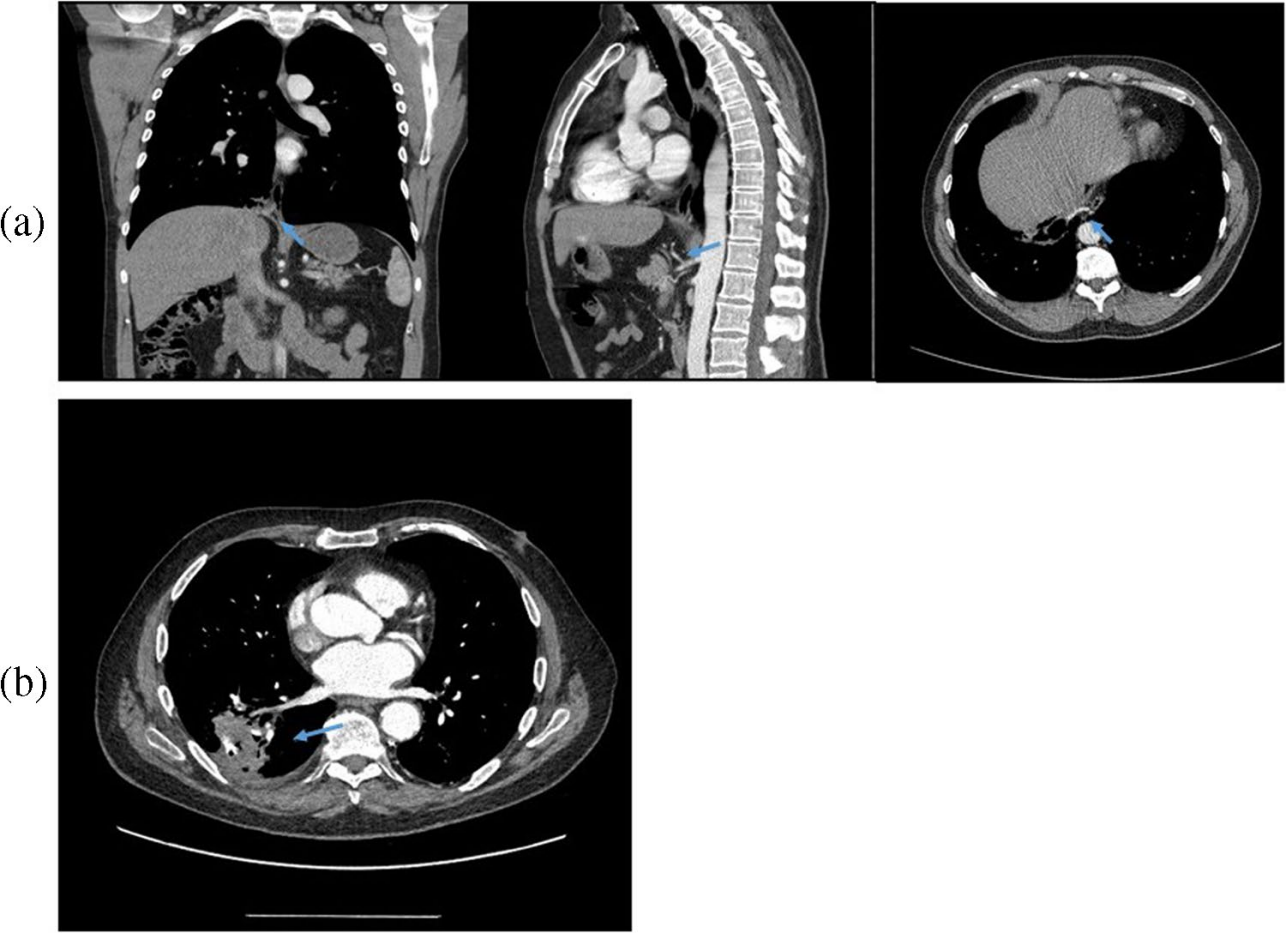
a A series of coronal, sagittal and axial contrast-enhanced computed tomography images of the thorax and abdomen identifying branches from the coeliac axis entering through the oesophageal hiatus to supply a small mass of the right lower lobe. b Contrast-enhanced CT image of the thorax showing a right lower lobe mass receiving blood supply from the pulmonary vein
